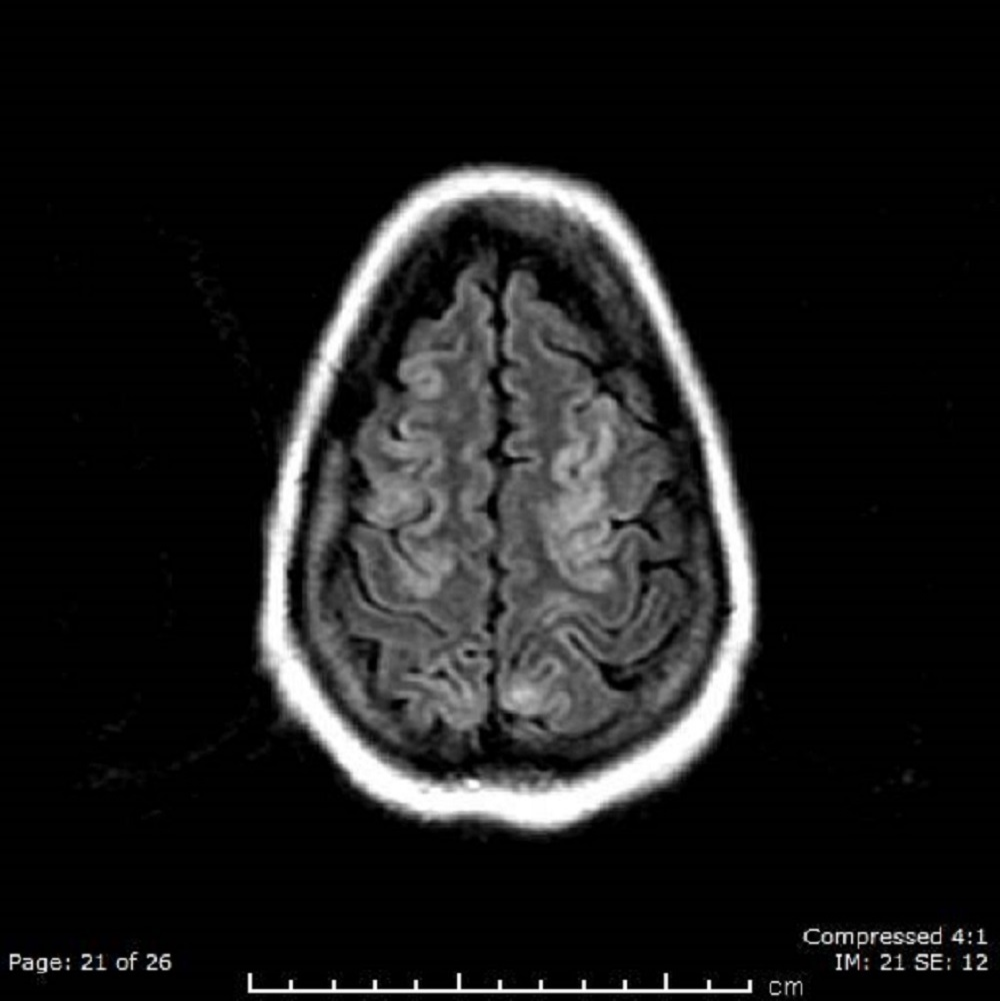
Brain MRI Shows Abnormal Signal at the Parieto-occipital Region
